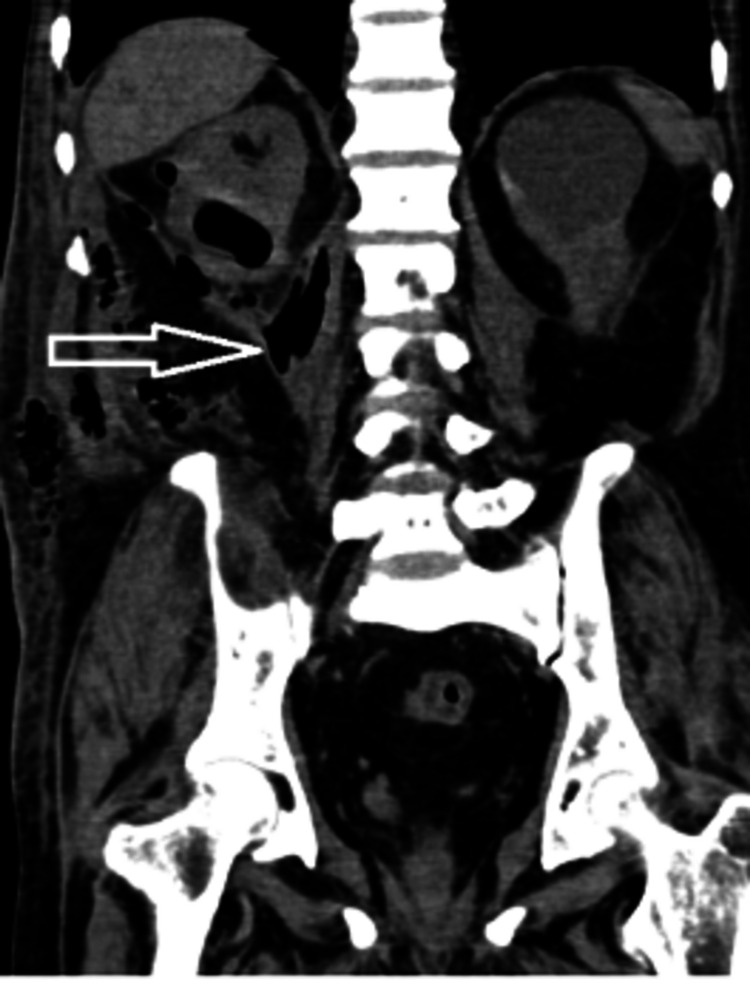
Contrast-enhanced CT abdomen coronal section showing a collection and air focus along the right psoas muscle CT: computed tomography
